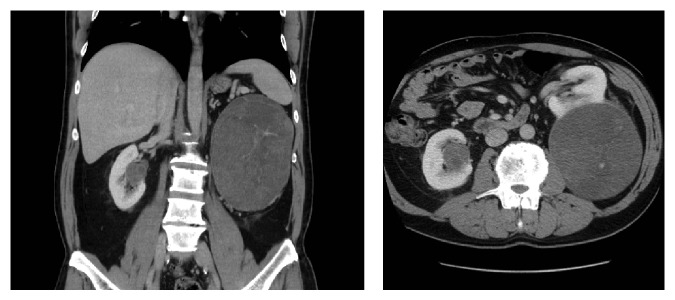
Infused axial and coronal CT abdominal images of patient 1.
